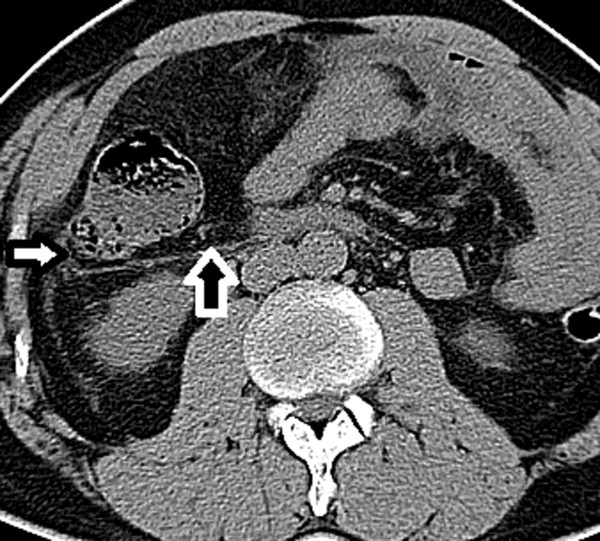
Transverse CT image of 46-year-old man with complicated appendicitis. Retrorenal plane, retromesenteric plane (black arrow), lateral conal plane (white arrow), subfascial plane, and bridging septa are involved.
